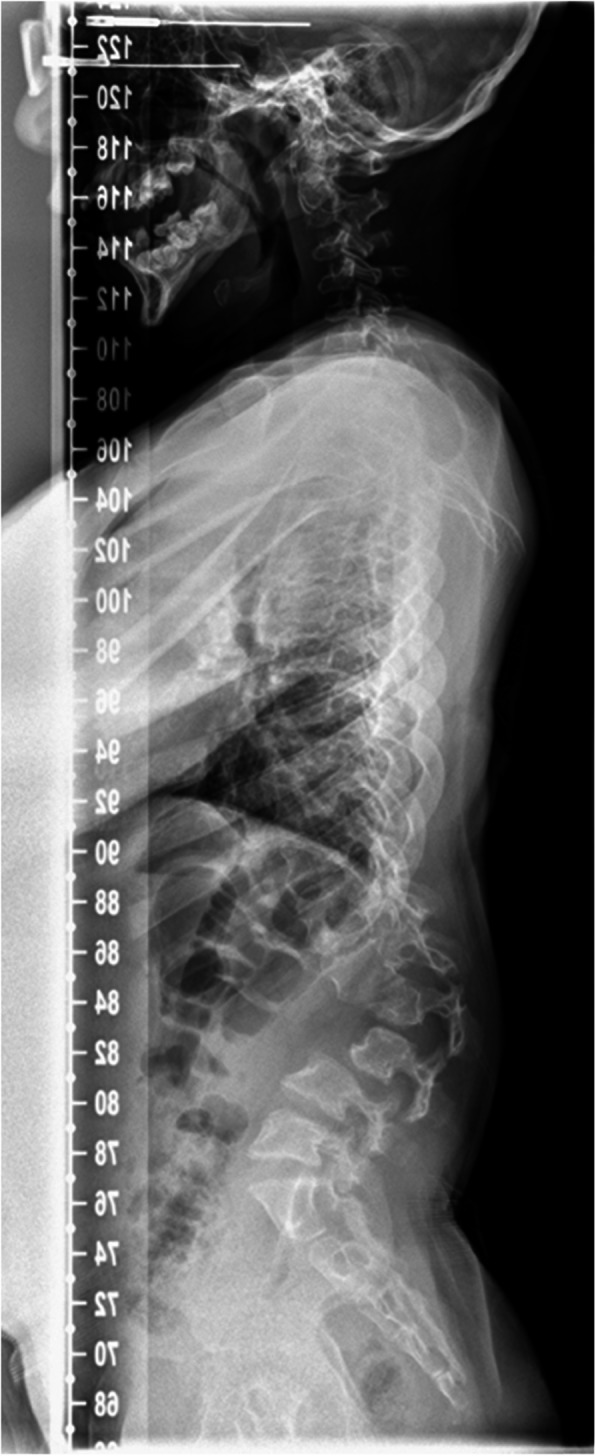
Pre-operative radiograph of thoracolumbar spine showing high lumbar kyphosis- Lateral view
